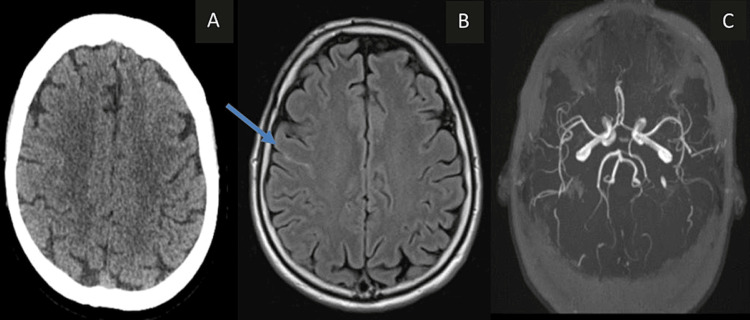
A 54-year-old man with sudden onset of left-sided paresthesia and facial numbness. (A) Unenhanced CT appears normal. There was no SAH. (B) FLAIR MRI on the same day shows focal SAH (blue arrow) in the right central sulcus. (C) MR angiogram was normal. Follow-up CT angiogram (not shown) was also normal CT: computed tomography; SAH: subarachnoid hemorrhage; FLAIR: fluid-attenuated inversion recovery; MRI: magnetic resonance imaging.
